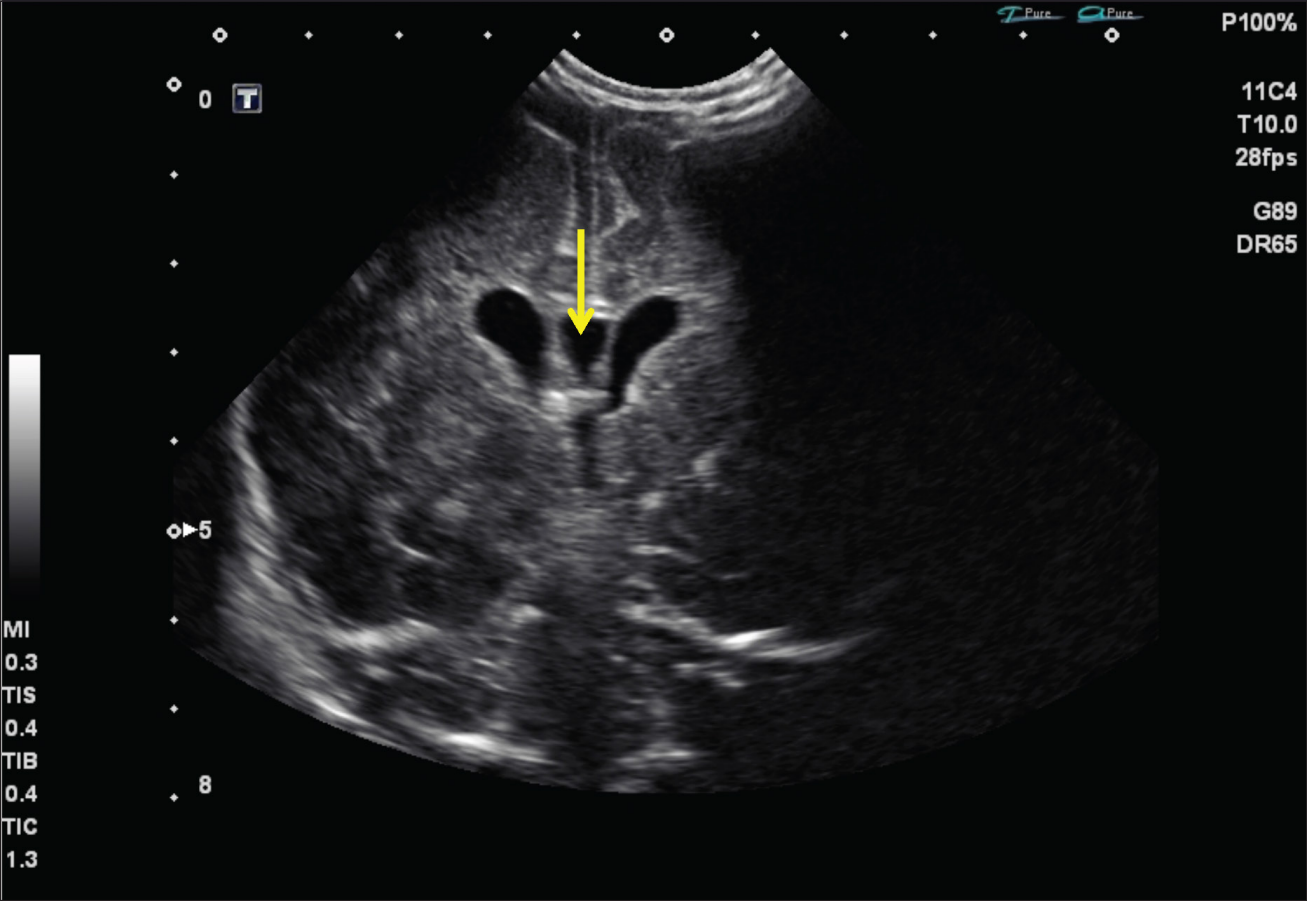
Transcranial ultrasound, day 16, coronal plane. Discrete enlargement of the third ventricle and lateral ventricles. Persistent cavum septum pellucidum, normal variant (arrow).
